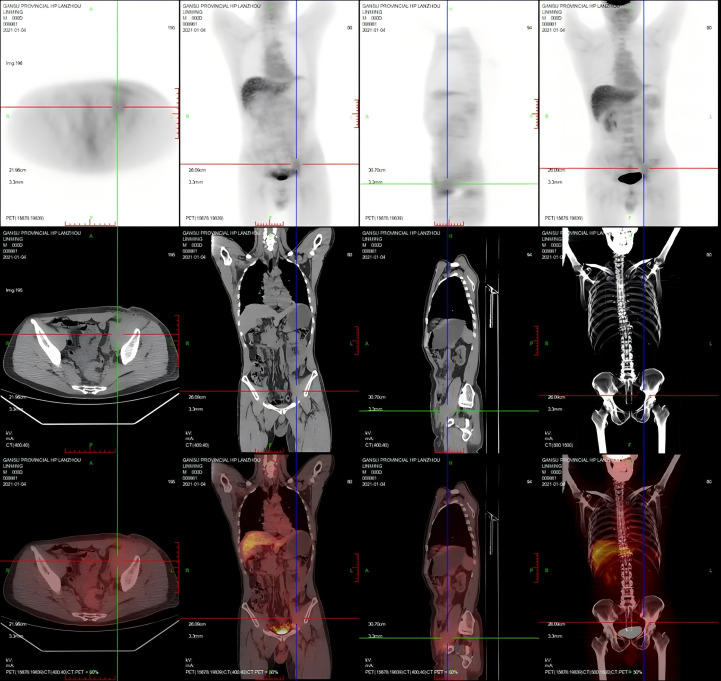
Baseline 18F-FDG PET/CT showing a peri-iliac perivascular soft-tissue lesion extending from the left common to external iliac vessels, with mild FDG uptake, compatible with myeloid sarcoma in the context of biopsy findings.
